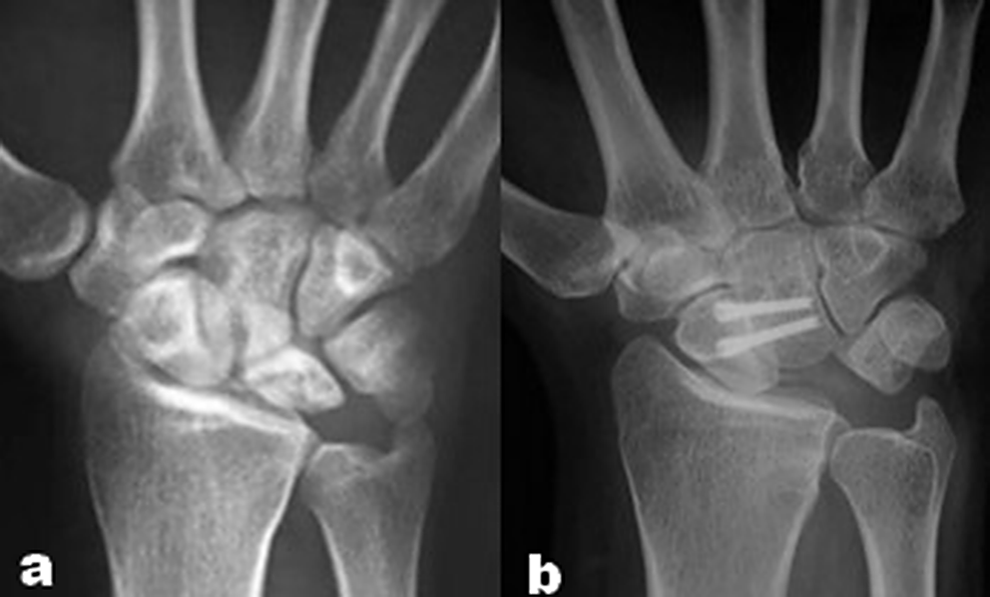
Posteroanterior radiographs of the wrist: (a) Preoperative image showing advanced osteonecrosis of the lunate consistent with stage IIIB Kienböck’s disease; (b) 18-month postoperative image demonstrating scaphocapitate arthrodesis with excision of the necrotic lunate, improved carpal alignment, and stable fixation
